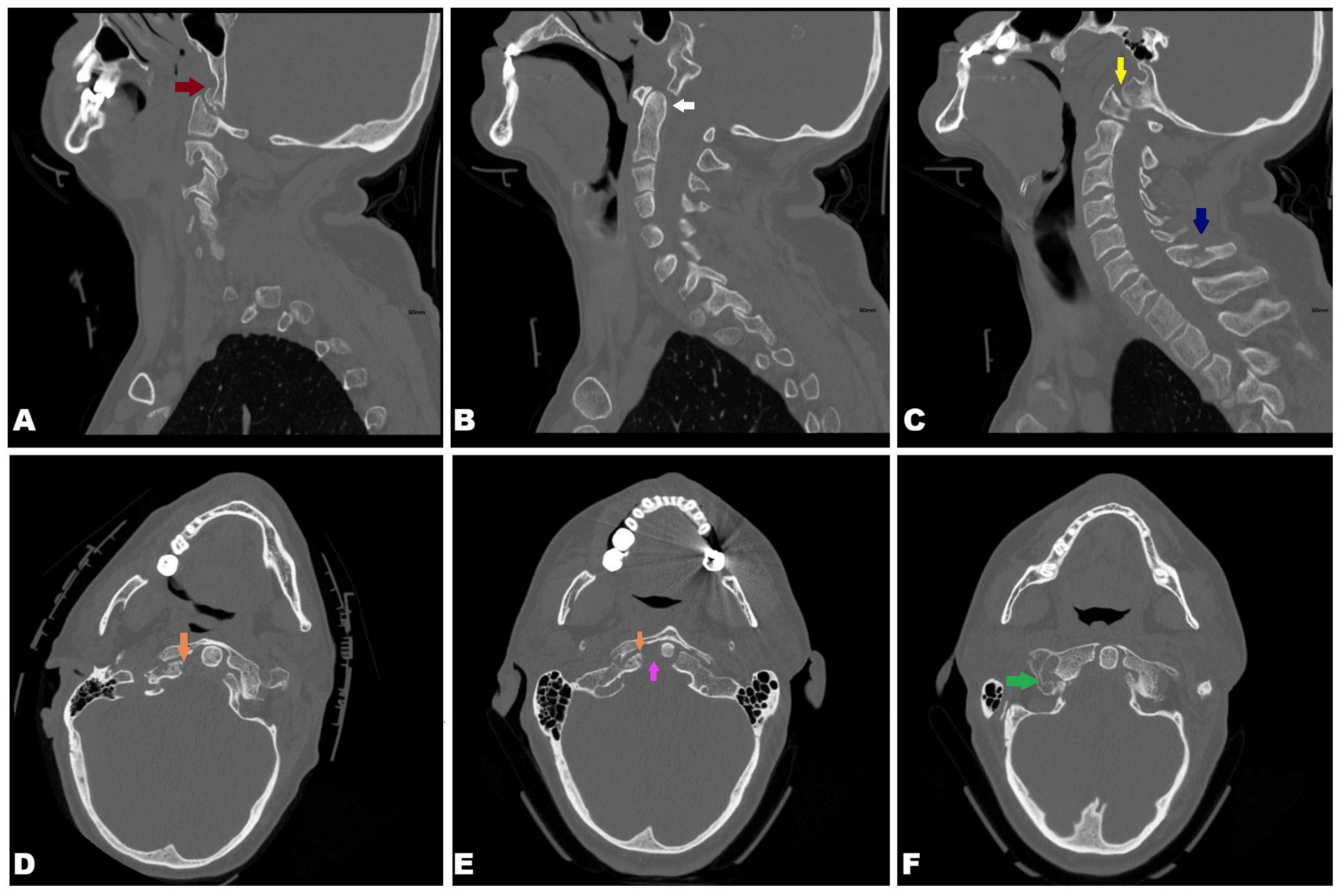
Sagittal (A, B, C) and axial (D, E, F) view CT images of the cervical spine demonstrating C7 fracture (subfigure C, blue arrow) and multiple previously missed injuries. Findings include a comminuted right occipital condyle fracture (subfigure D and E, orange arrows), a right C1 lateral mass fracture extending into the right foramen transversarium (subfigure F, green arrow) with associated impaction on the right occipital condyle (subfigure A, red arrow), bilateral widening of the atlanto-occipital joint spaces (subfigure C, yellow arrow), widening of right odontoid lateral mass interspace (subfigure E, pink arrow) and atlanto-occipital subluxation (subfigure B, white arrow). CT: computed tomography
